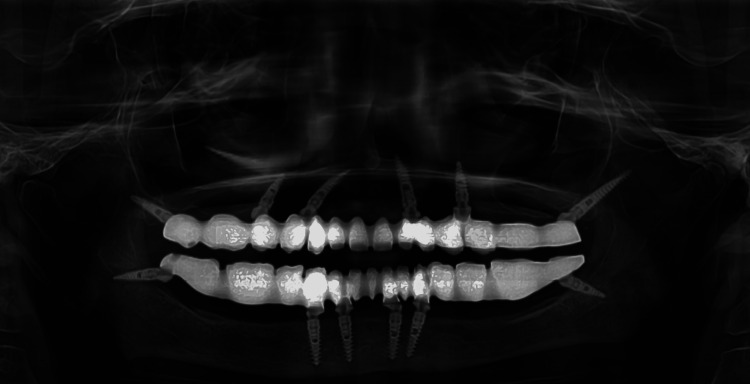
Orthopantomogram of the patient after prosthesis placement
